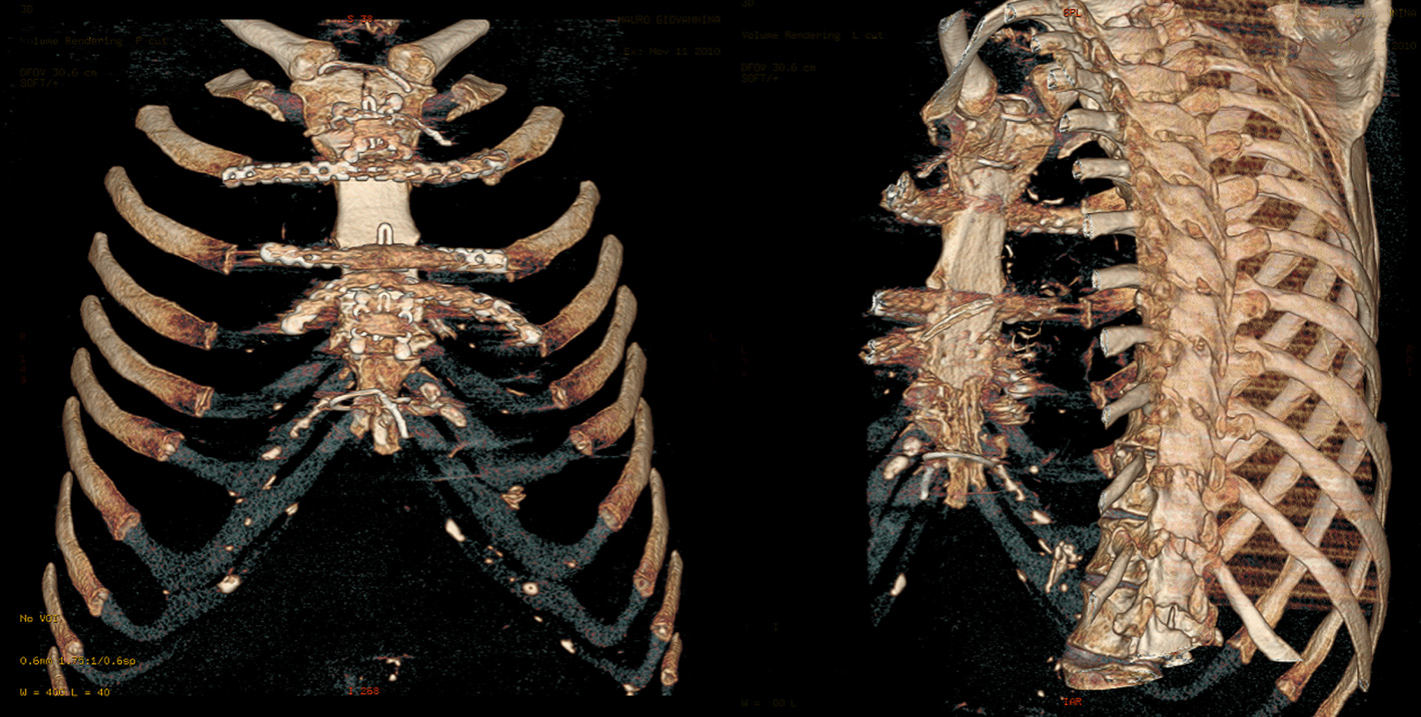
CT scan reconstruction 2 months after surgery. The transplanted sternum appears well integrated with the rib cage.
